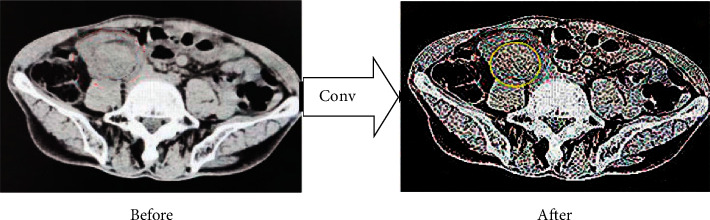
The processed pelvic CT image.
